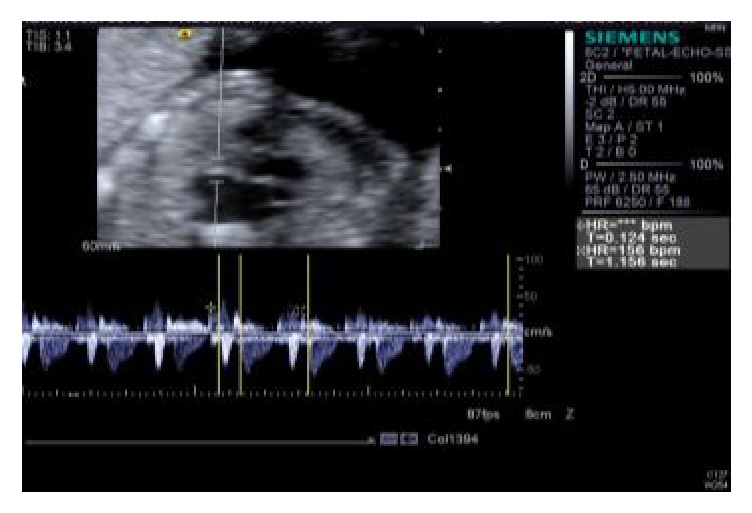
Normal mechanical PR interval (mPR) 10 days earlier in our case; fetal cardiac Doppler sampling the mitral inflow to aortic outflow.
